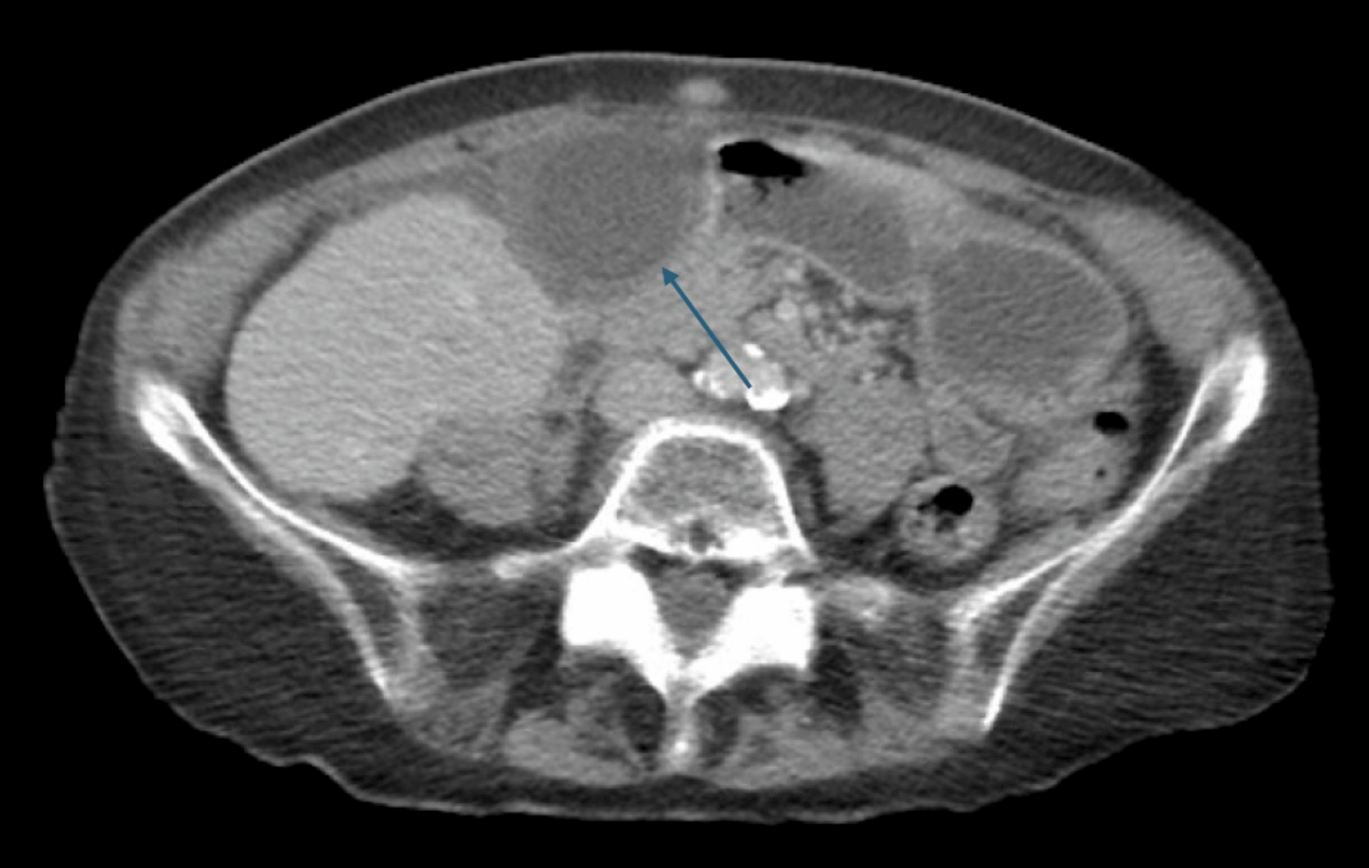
CT scan showing pericholecystic fluid, gallbladder wall thickening, and edema The blue arrow highlights the gallbladder with notable wall thickening and surrounding pericholecystic fluid and edema. CT: computed tomography
